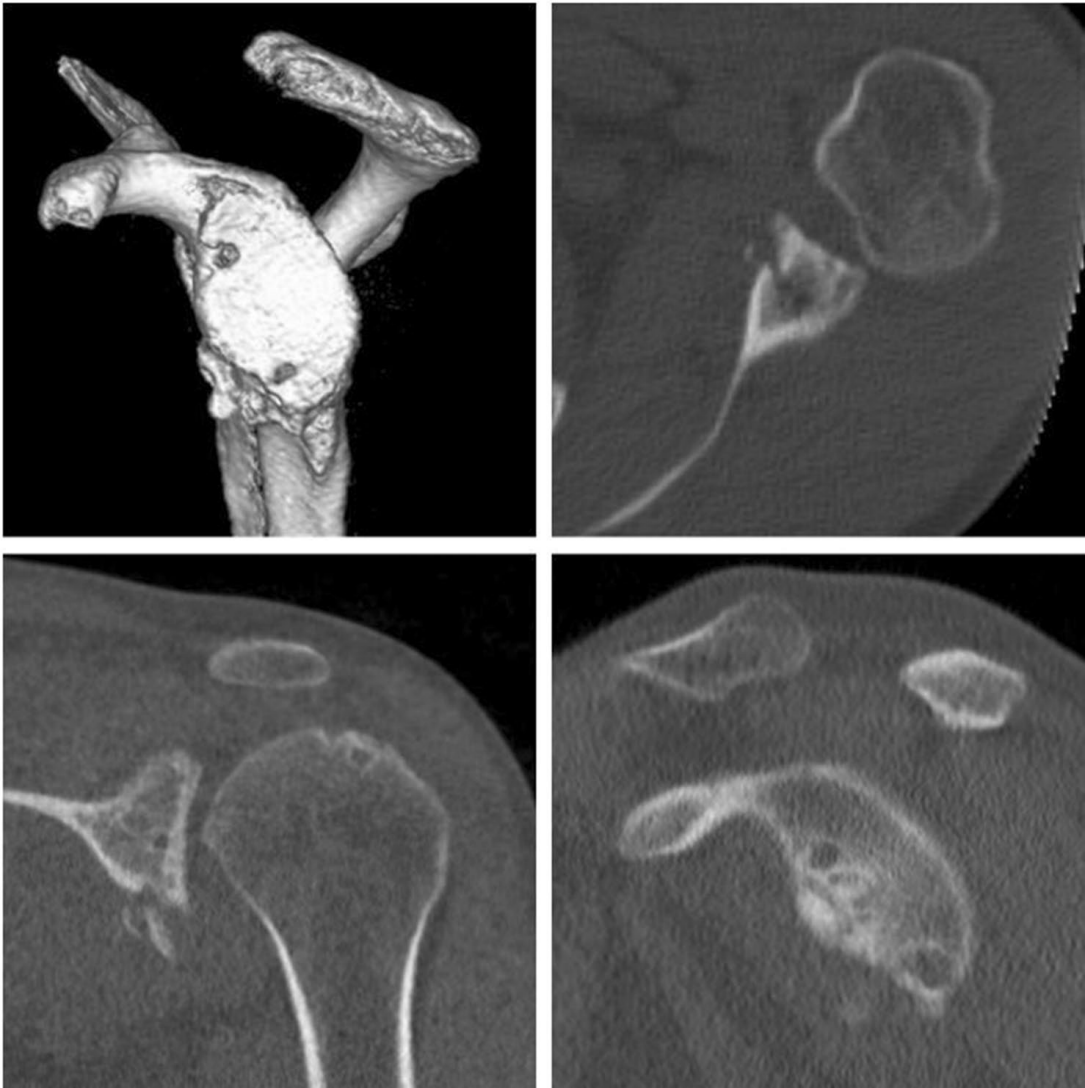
CT scan at 68 months postoperative of the patient who experienced a traumatic recurrent dislocation with a fracture of the former iliac crest bone graft
